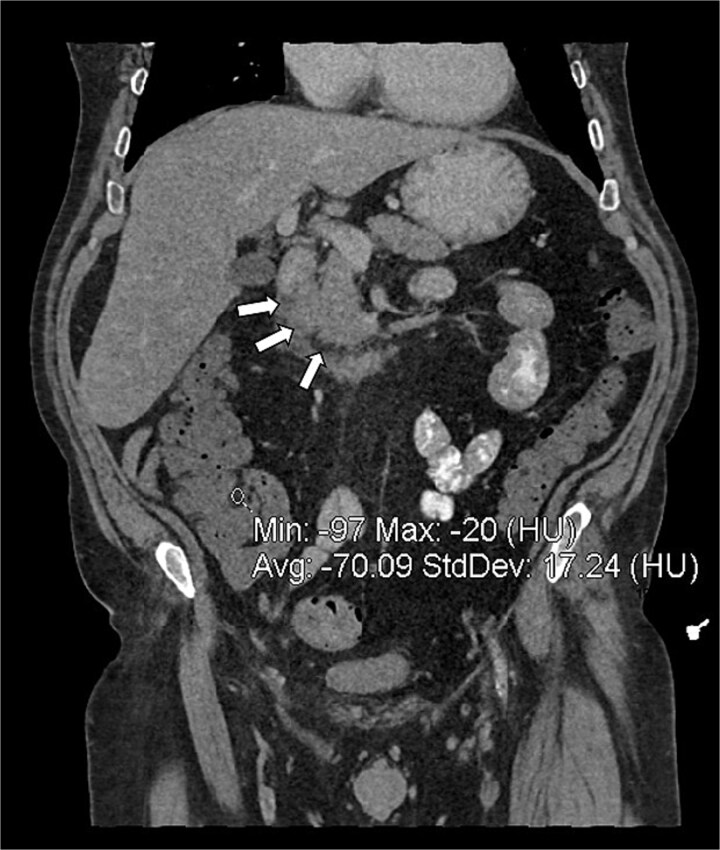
Coronal reconstruction of abdominal CT showing inflammatory fat stranding (arrows) in the pancreaticoduodenal groove, between the duodenum and the uncinate process of the pancreas.
